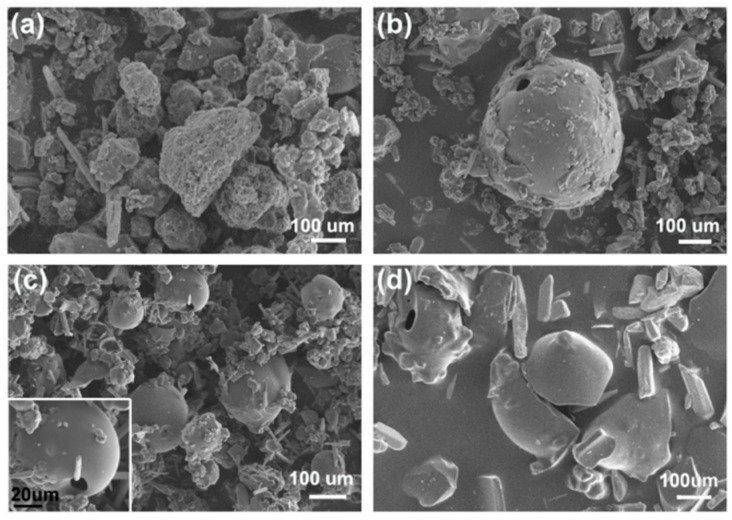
SEM images of (a) control and (b-d) lignin-based carbon materials in 40 wt % ZnCl_2_ solution using (b) microwave, (c) ultrasound, and (d) UV irradiation, respectively.
